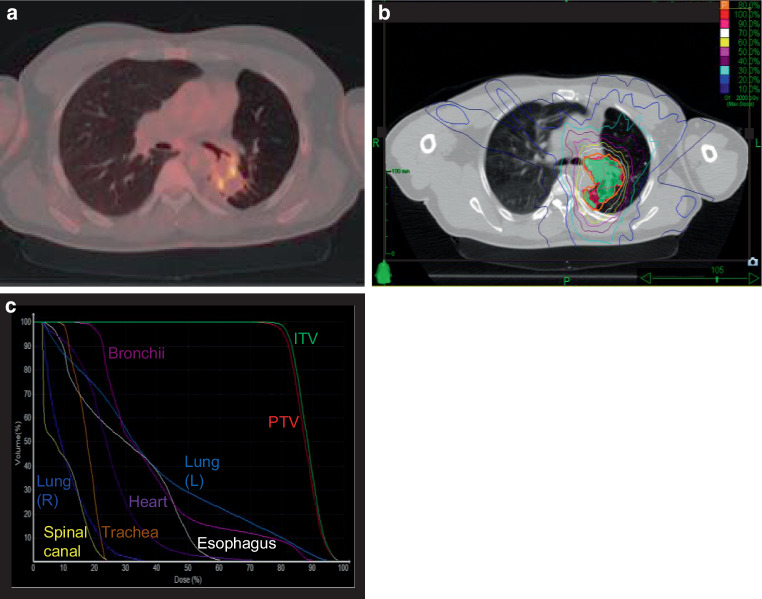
Treatment planning and modalities including dose–volume histogram (DVH). a Treatment planning included an ^18^F‑FDG-PET/CT scan to precisely localize the fungal manifestation and the primarily inflamed and eroded vascular lining of the cavity, most likely causing the bleeding. b 16 Gy was applied in two fractions of 8 Gy on consecutive days with a robotic arm-mounted linear accelerator. Dose was prescribed to the 80% isodose line. PTV (*red*) was generated from an ITV (*green*) by adding a 2-mm margin. The ITV accounts for motion of the target lesion during respiration registered by a 4D planning CT. The PTV on the other hand accounts for setup and planning uncertainties. c The DVH represents the exact radiation dose (shown as percentage of the prescribed dose) delivered to the target volumes and organs at risk (OAR; also shown as percentage of total volume). *CT* computed tomography*, CTV* clinical target volume*, FDG* fluorodeoxyglucose*, ITV* internal target volume*, PET* positron emission tomography*, PTV* planning target volume*, RT* radiotherapy
